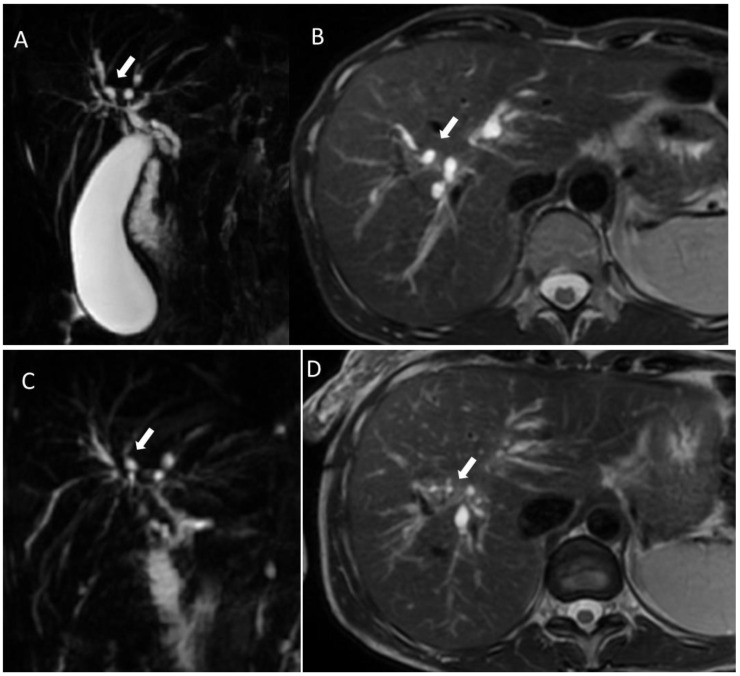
MRI of a 45-year-old patient affected by sclerosing cholangitis at baseline ((A): cholangiography sequence; (B): T2-weigthed sequence in axial plane) and 6 years later after cholecystectomy ((C): cholangiography sequence; (D): T2-weigthed sequence in axial plane). (A,C) 3D Magnetic resonance cholangiopancreatography and (B,D) T2 Axial images. The figures show progressive strictures and focal dilatations (white arrows) of intrahepatic biliary ducts over a period of 6 years.
